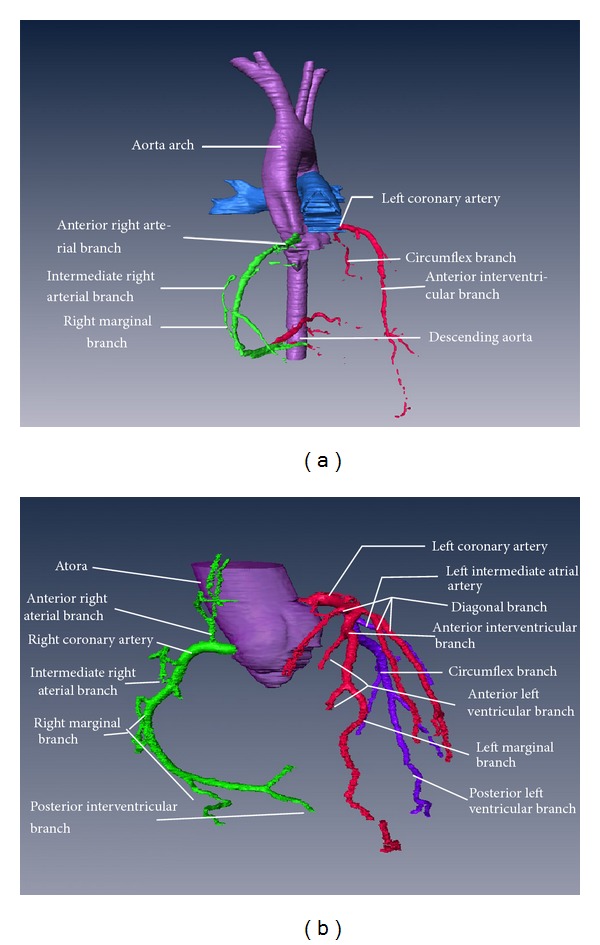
3D visible models of the coronary arteries based on the CVH and cardiac CT datasets. (a) Coronary artery model based on the CVH dataset. (b) Coronary artery model based on the cardiac CT dataset.
